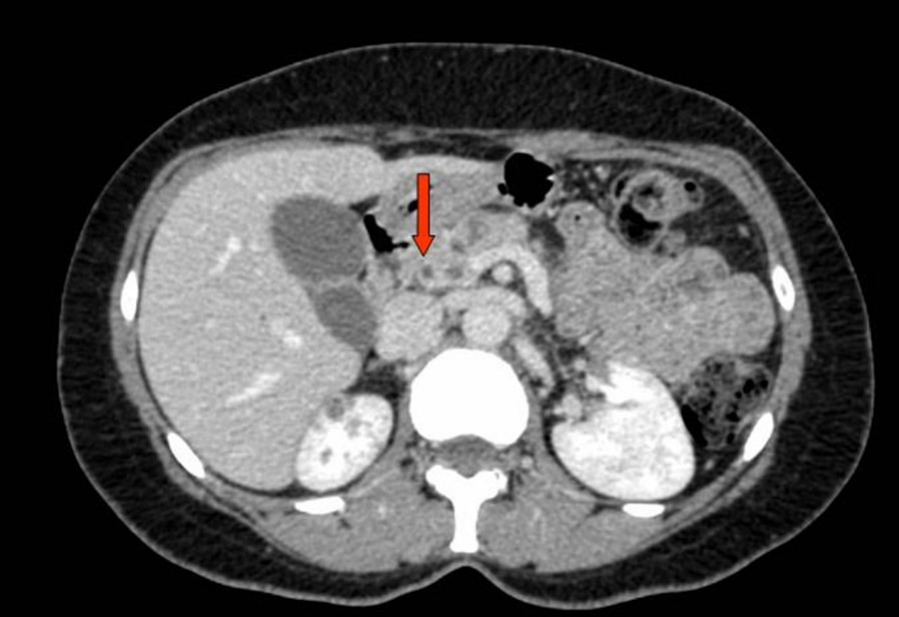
Contrast-enhanced CT-scan with detection of multiple hypodense pancreatic lesions
